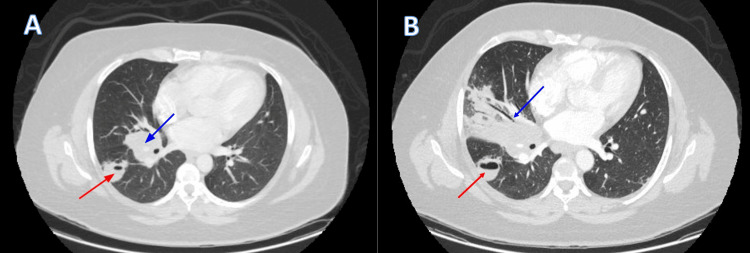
Initial computerized tomography (CT) scans Presence of right hilar lobulated mass measuring 4.5*4.5 cm (blue arrow) and RLL cavitary lesion measuring 2.8*3 cm with air-fluid level (red arrow) in soft tissue window (A) and lung window (B).
